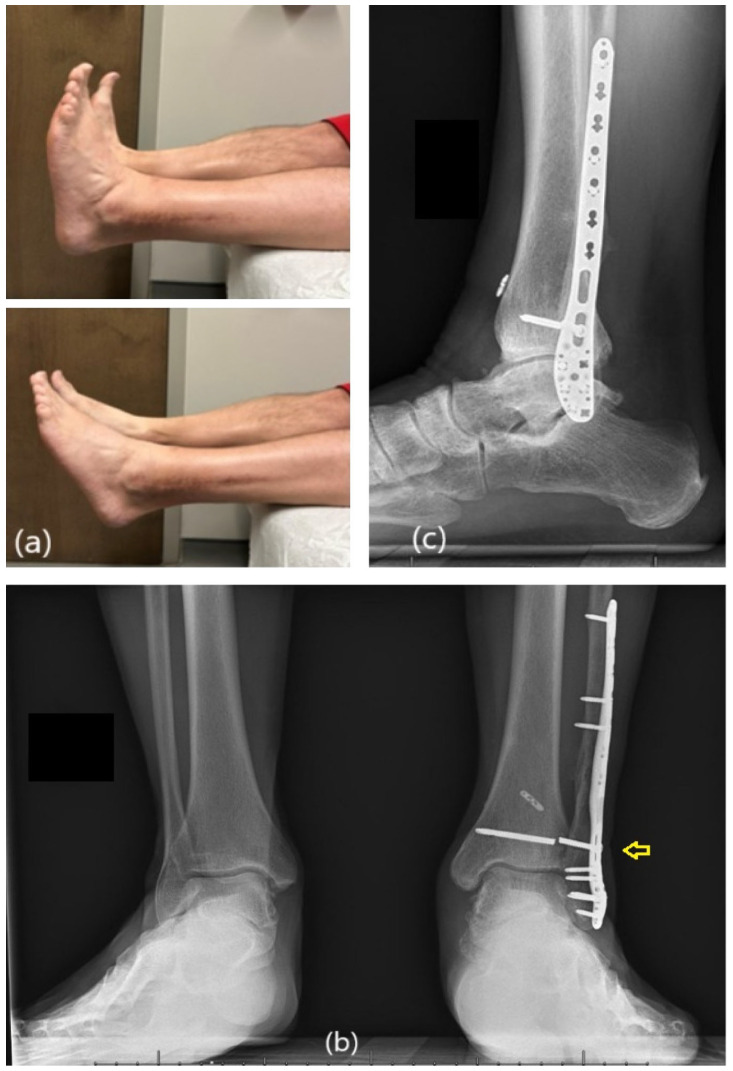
Final 3-year follow-up. (a) Clinical images showing functional ankle motion. Weight-bearing AP (b) and lateral (c) radiographs showing broken syndesmotic screw (yellow arrow) with congruent ankle mortise.
